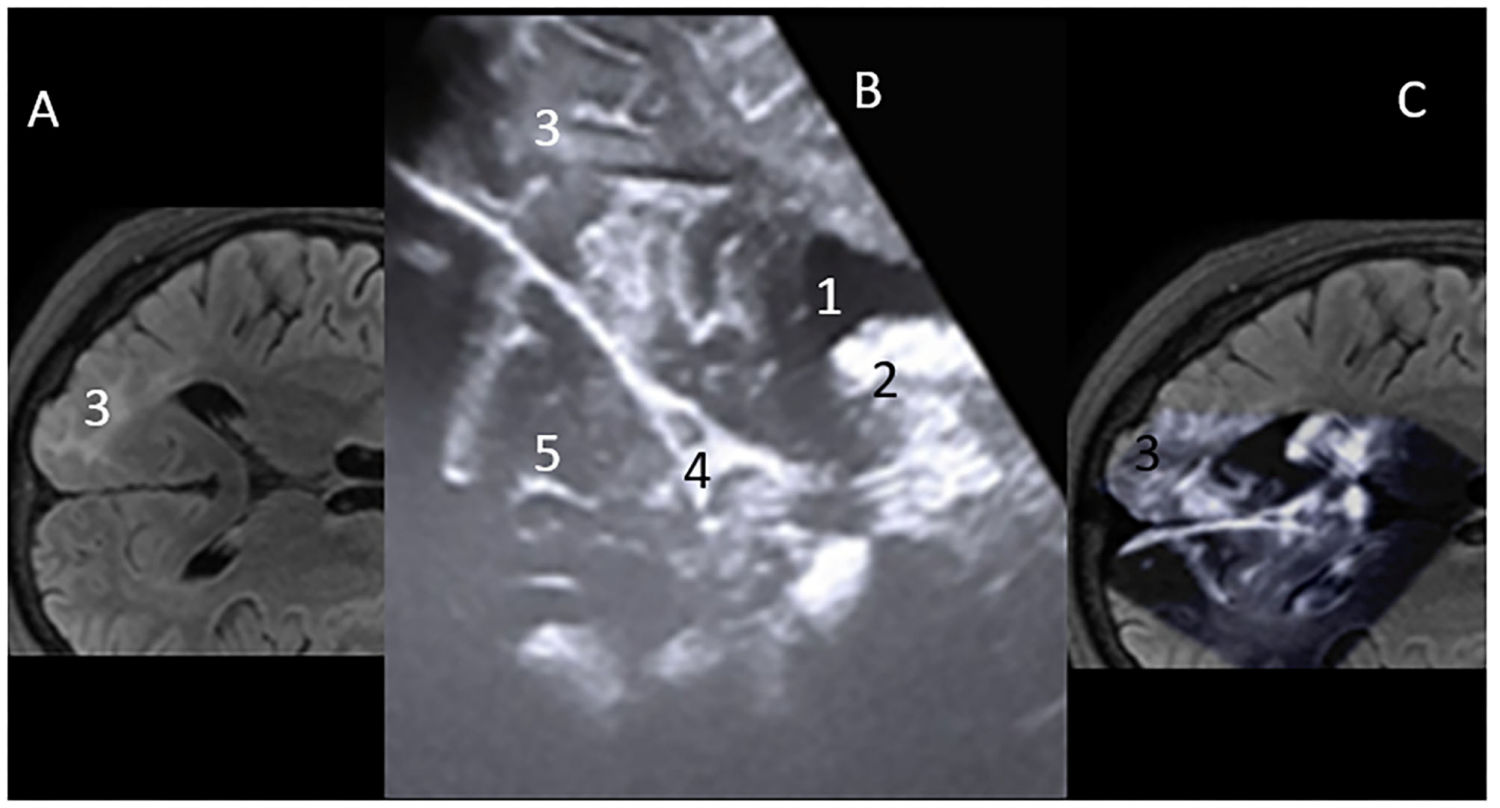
(A) MRI – flair axial view of Rt occipital lobe Focal cortical dysplasia (FCD) lesion-3. (B) Mirror iUS Axial view of Rt occipital lobe FCD lesion-3. Cerebral spinal fluid (CSF) in ventricle occipital horn is “Hypoechoic” on iUS-1; “Hyperechoic” futures vascular stuctures, high grade tumors. Here, the choroid plexis is hyperechoic-2. Falx- 4. Normal brain tissue on iUS is “isoechoic”-5. (C) Projection on the intraoperative Ultrasound Concordance of lesion location on MRI and iUS.
